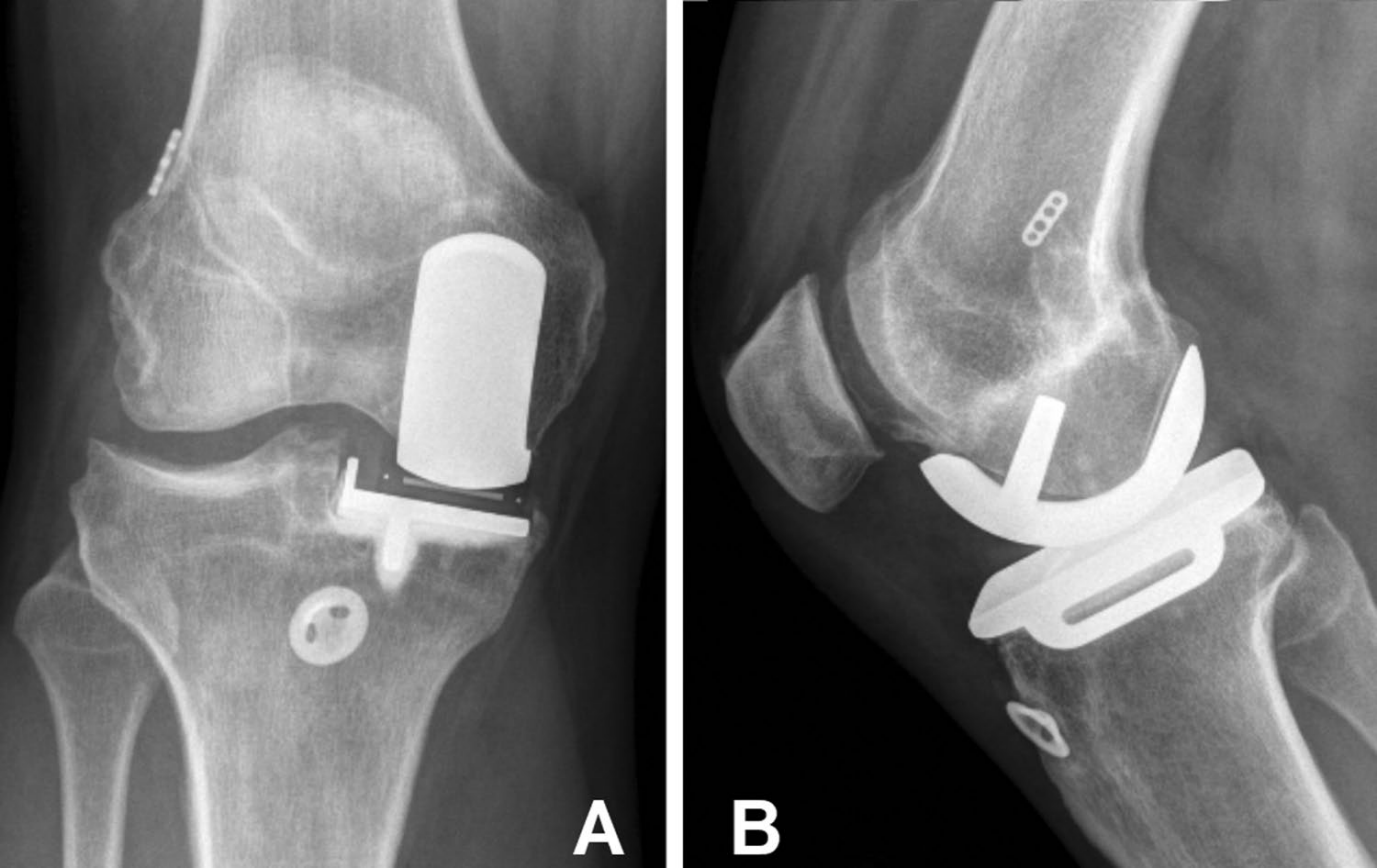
Conventional radiographs with anterioposterior (A) and lateral views (B) in a 54 year-old patient 10 years postoperatively after unicompartmental knee arthroplasty and anterior cruciate ligament reconstruction of the right knee
